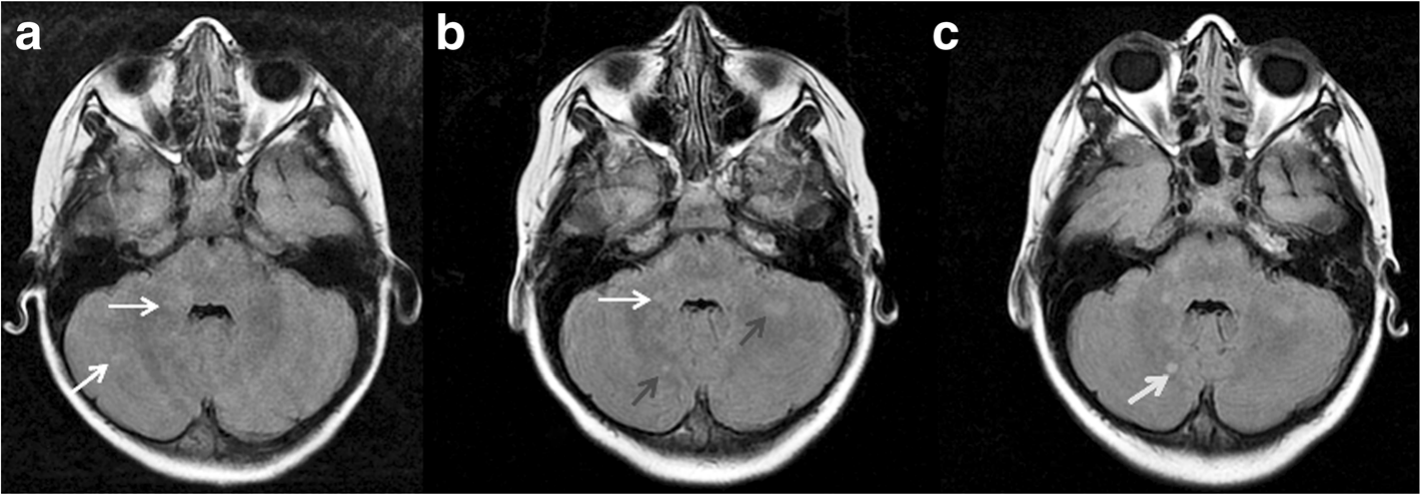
The figure shows pattern D (see text) on axial MRI in a patient with NF1. Two small right cerebellar abnormal signal intensities (arrows) are seen at 8 years (a). New right and left cerebellar abnormal signal intensities (dark oblique arrows) developed at age 11 years. One of the older abnormal signal intensities is also shown (horizontal arrow) (b). The right cerebellar abnormal signal intensity increased in size (arrow) at age 13 years (c)
